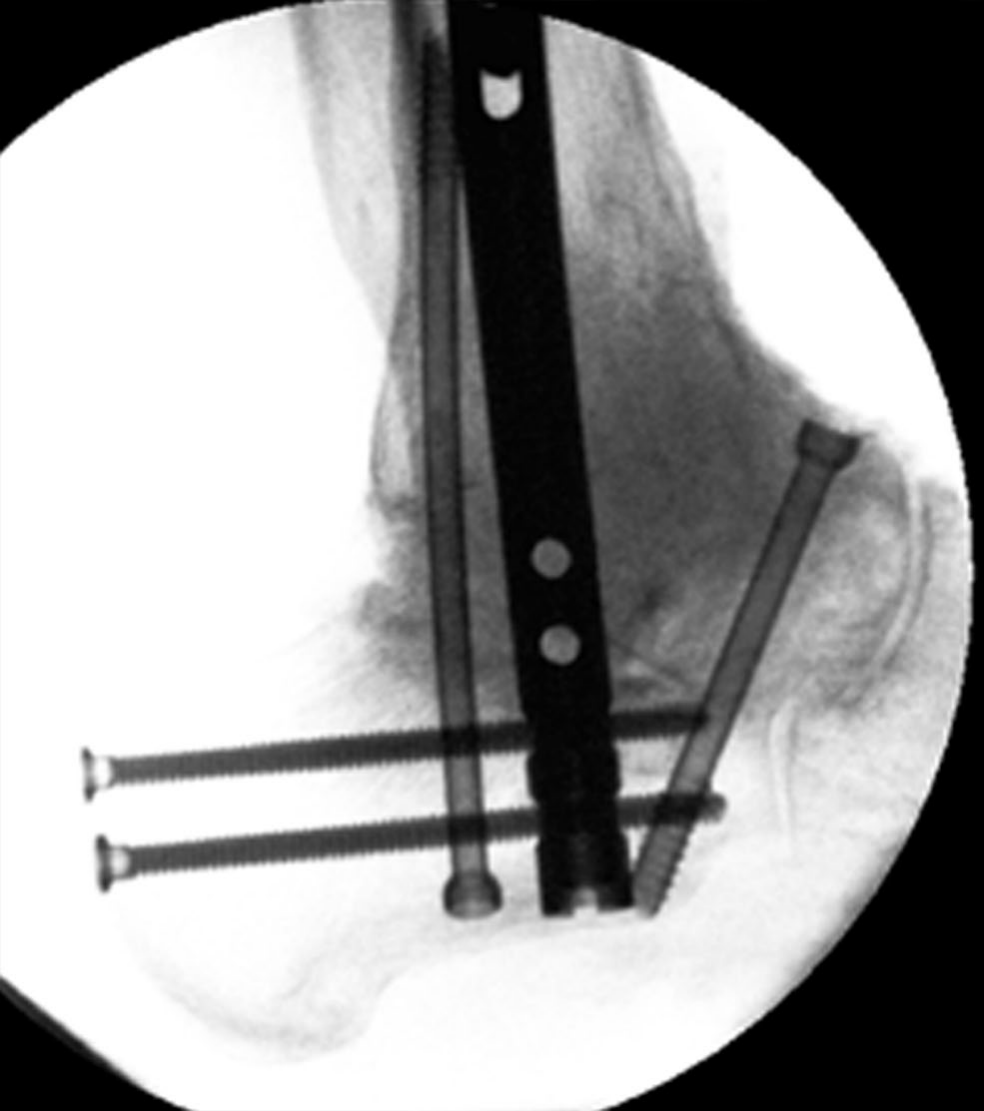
Intraoperative lateral radiographs of the case from Figure 1 showing the appearance on intraoperative imaging and the extent of bone graft impaction through the talus and tibia. In this case, an extra screw was used to secure the talar head to the construct as the talar head had poor bone stock.
